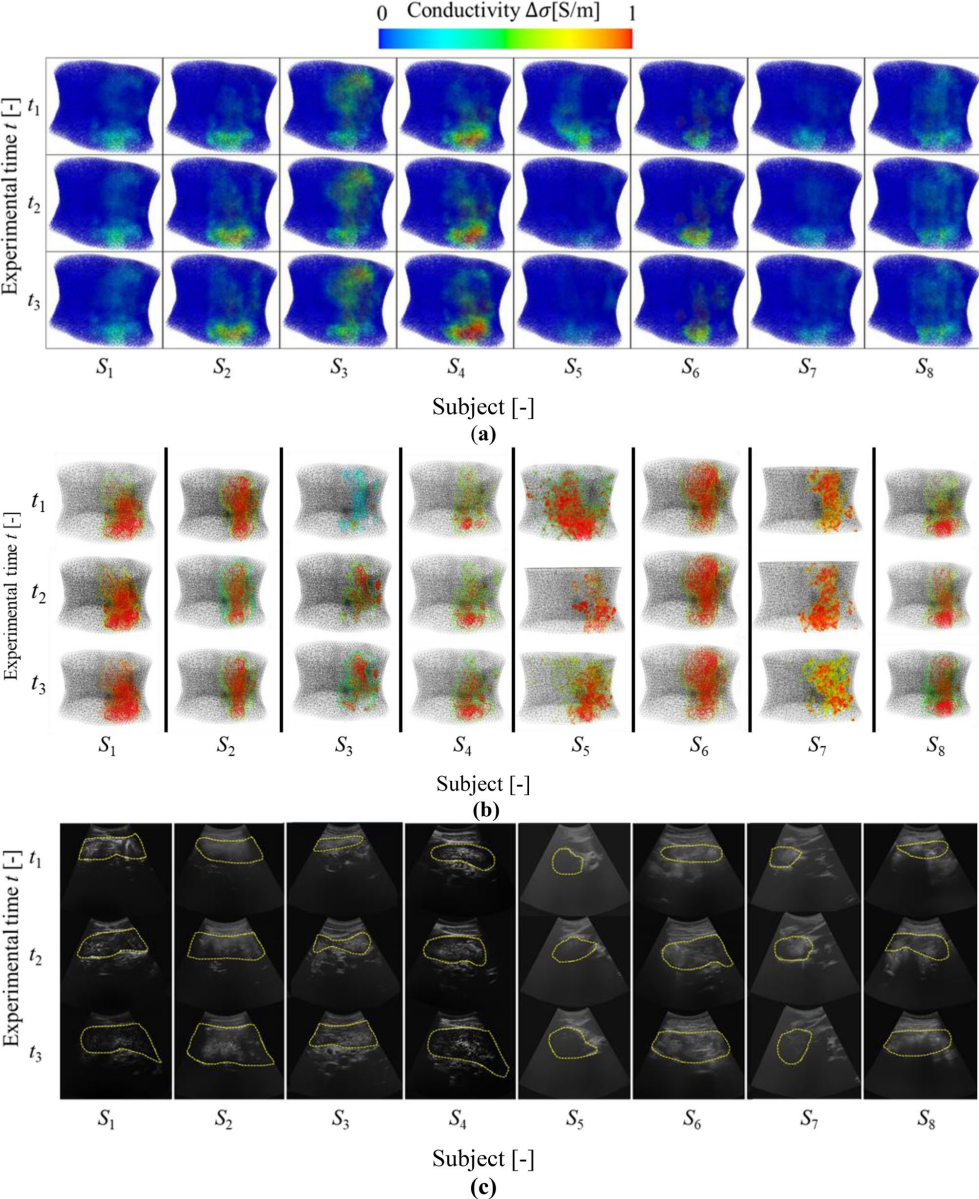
(a) Visualization of gastric conductivity distribution σ by *g*EIT Suit, (b) Extraction of clustered gastric conductivity distribution ^*k*^σ by fuzzy clustering, (c) Evaluation of gastric area by ultrasound.
